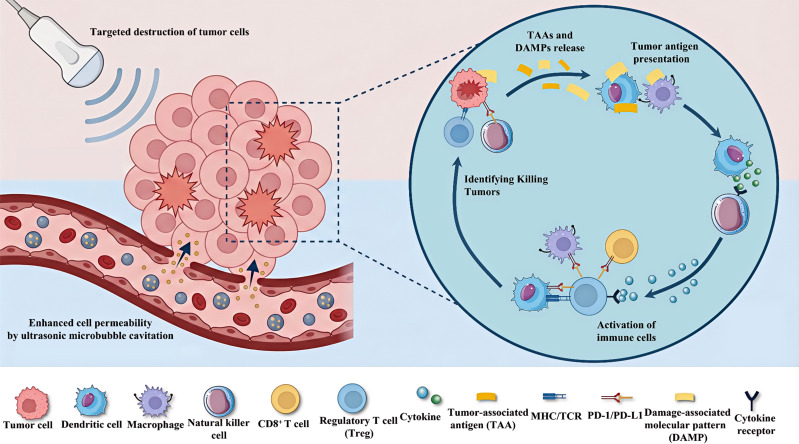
Ultrasound–microbubble synergy in tumor immunotherapy (created with biorender.com).
